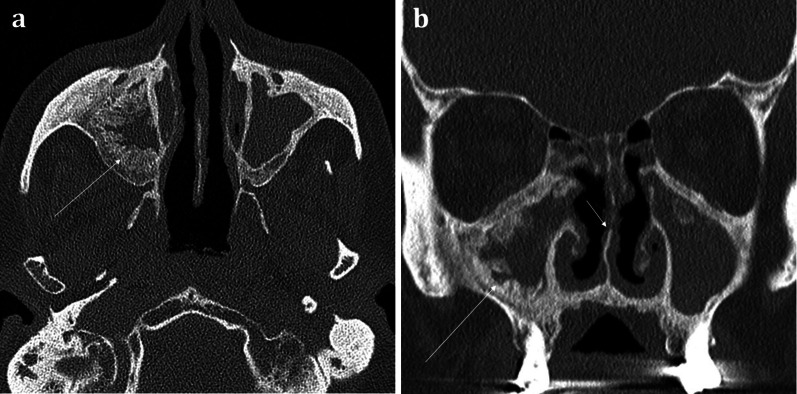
Axial (a) and Coronal (b) bone window CT images of a 71-year-old patient with known granulomatosis with polyangiitis (GPA) suffering from chronic nasal obstructive symptoms and nasal crusting. There is widespread mucosal thickening throughout the sinuses with evidence of “frond-like” chronic remodelling and osteitis (long arrows). On the coronal image (b), erosion of the middle turbinates and irregularity of the nasal septum can be seen (short arrow)
